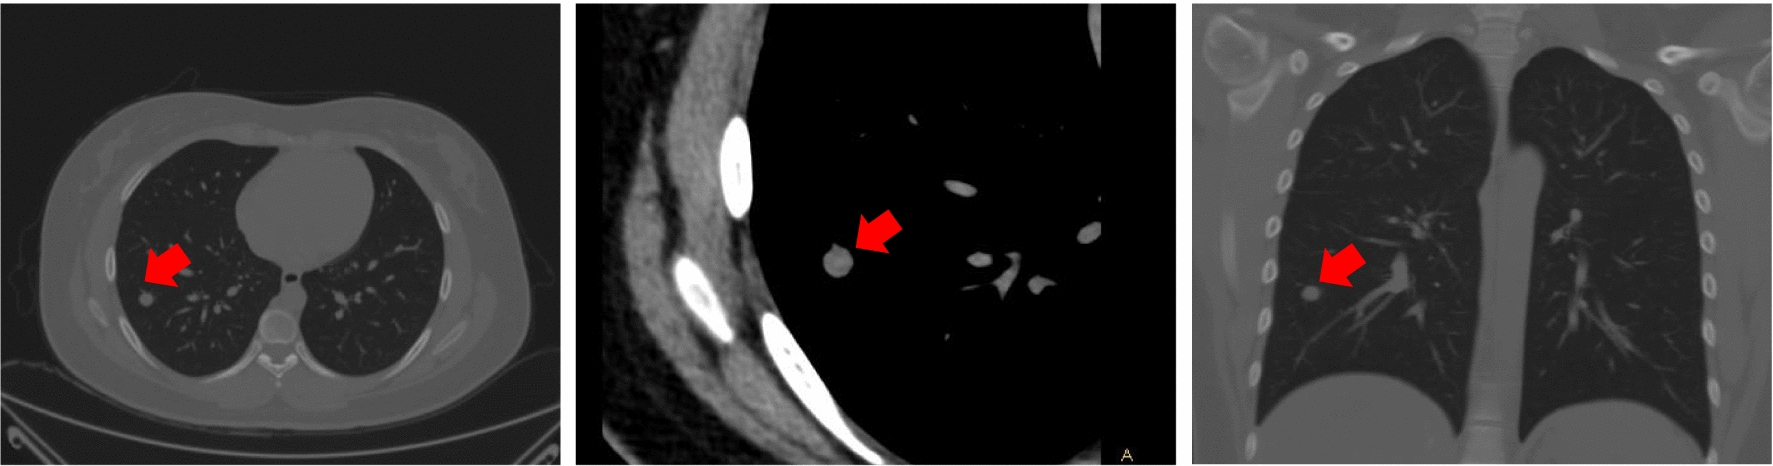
Transverse section and coronal section of CT scans indicated occupied lesion located in right lung, which were labelled as red arrow. The pathological diagnosis was neuroendocrine tumor (NET) carcinoid
